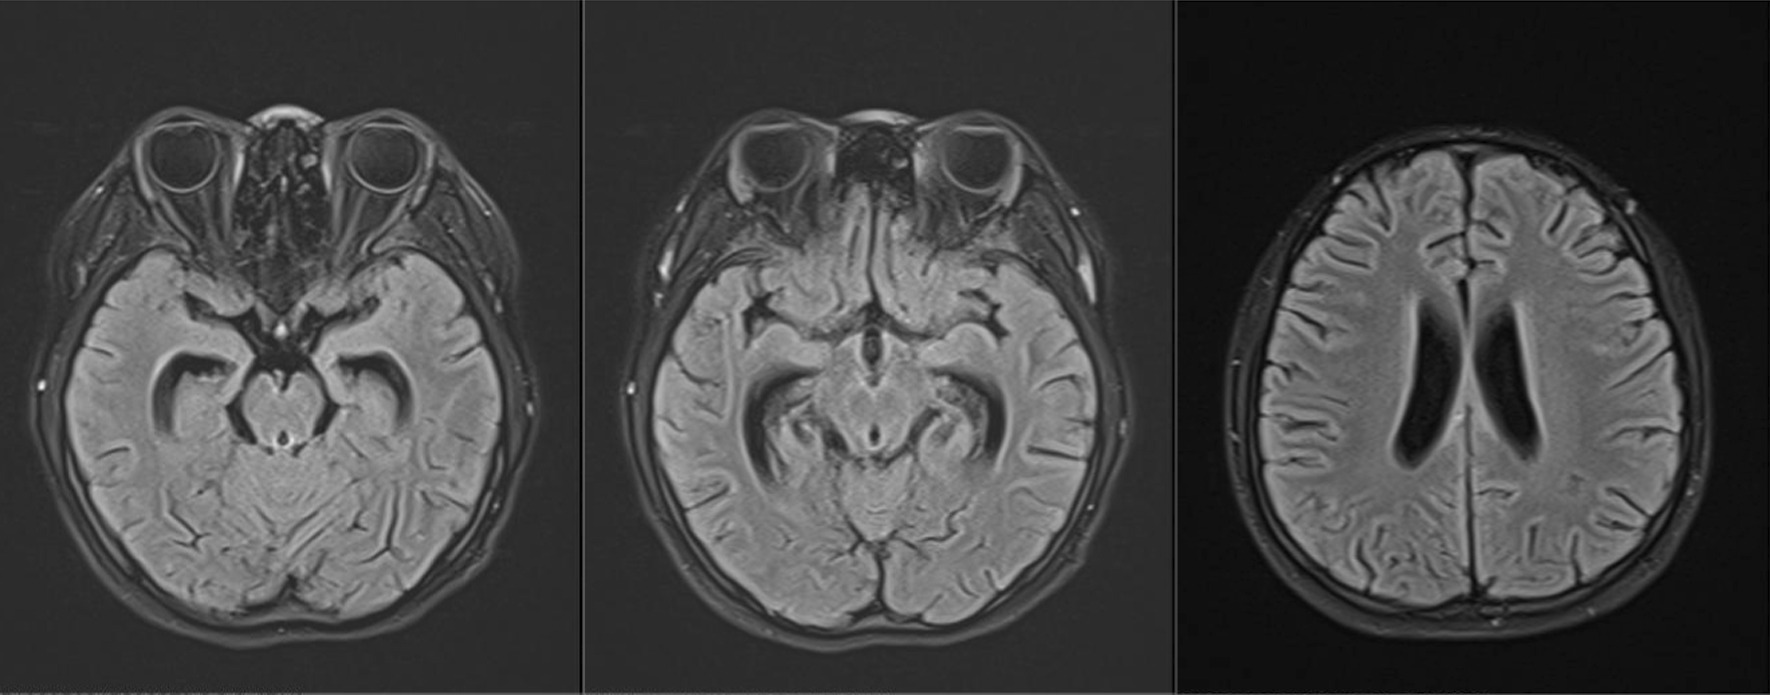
The cranial enhancement MRI showed punctiform enhancement in the pontine brain, right cerebellar hemisphere, bilateral frontal, temporal and parietal lobes, nodular enhancement in the local meninges, and linear enhancement in the brain pool
